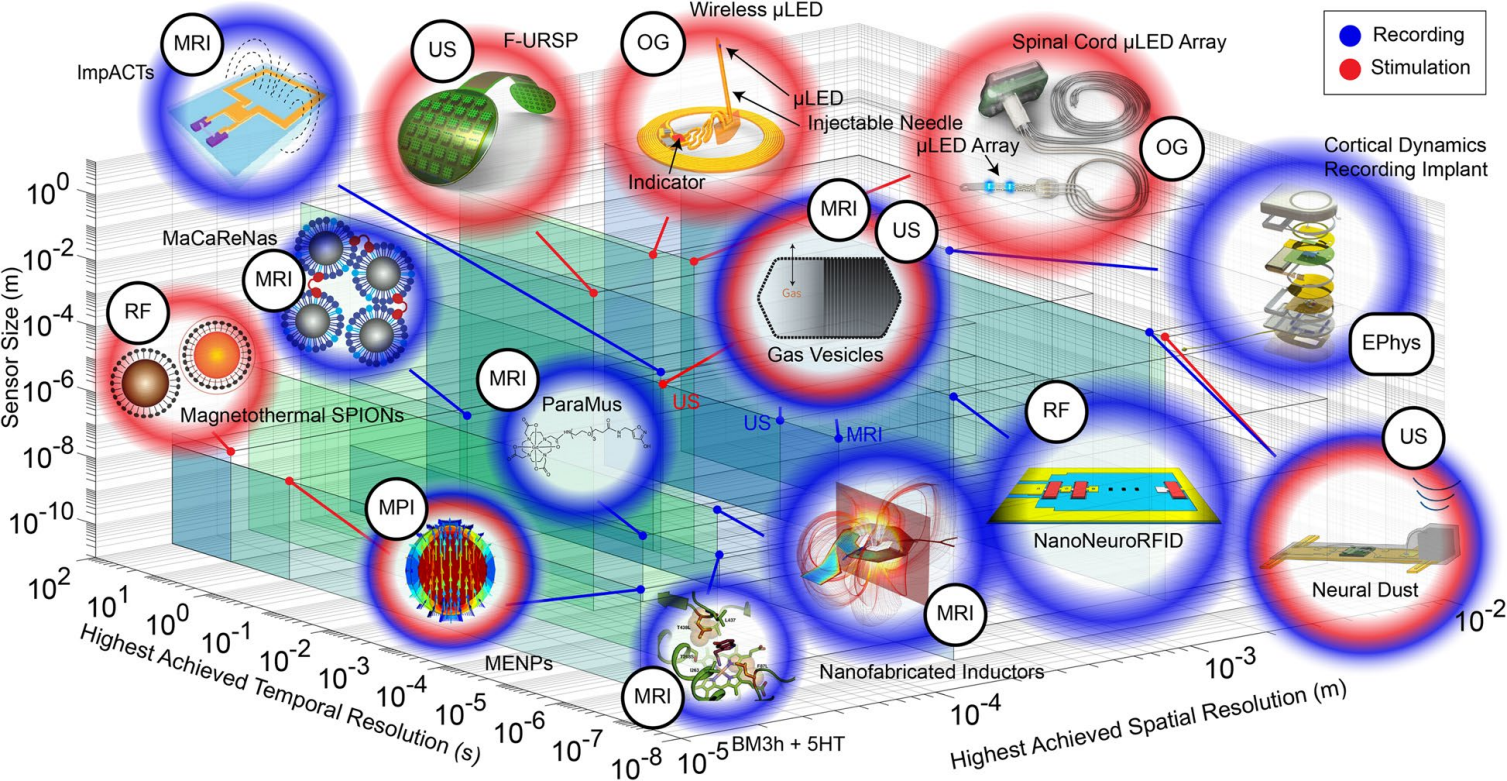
Overview of the approximate sizes and spatiotemporal resolutions of neural transducers. Blue halos indicate probes used for recording, red halos indicate stimulation and bicolor halos indicate dual recording/stimulation capability, smaller black circles indicate corresponding imaging techniques (MRI = magnetic resonance imaging, MPI = magnetic particle imaging, US = ultrasound, EPhys = electrophysiology, RF = radiofrequency, OG = optogenetics). Tinted blue-green planes are included to facilitate context determination of spatial resolution (x-axis), temporal resolution (y-axis), and sensor size (z-axis). See the corresponding data in Table 1 for precise values
